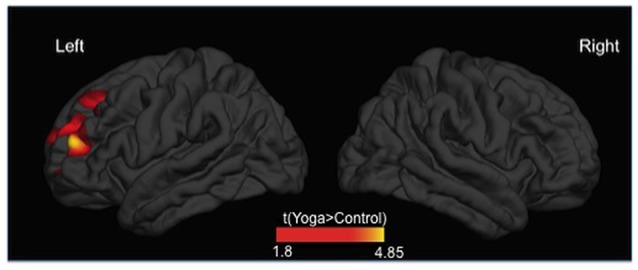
Differences in cortical thickness (CT) between yoginis and controls (*P* < 0.05, cluster corrected). Yoga practitioners showed greater CT in a cluster in the left prefrontal cortex (RTF-based, cluster-corrected, *p* < 0.05).
